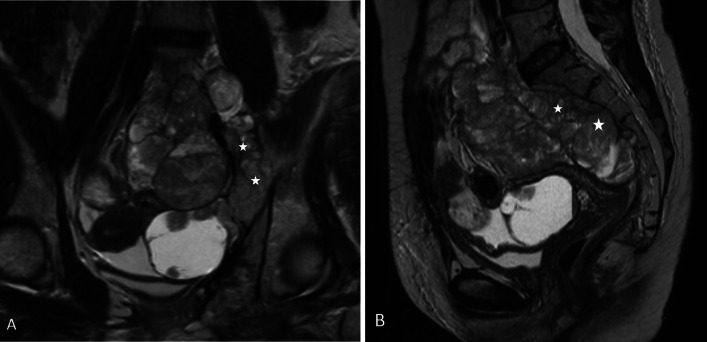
a, b MRI images of a patient with biphasic malignant peritoneal mesothelioma. a Coronal MRI of the pelvis shows a large mixed solid cystic mass in the pelvis involving the left pelvic side wall (*). b Sagittal MRI of the pelvis shows the mass extending into presacral space (*). These constitute U2 findings which render complete cytoreduction unlikely
